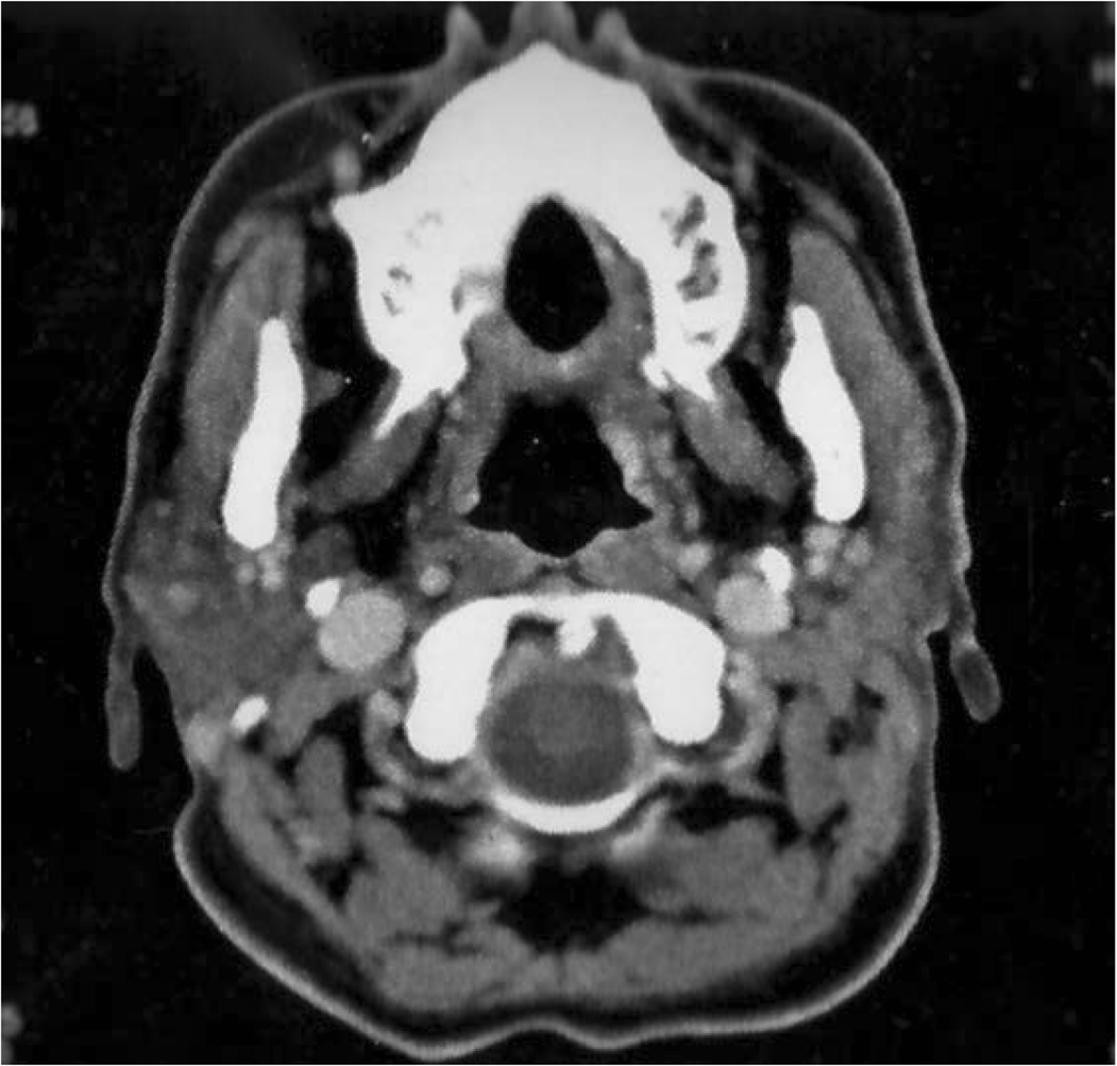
Postoperative computed tomography scan showing no residual tissue in parotid space
